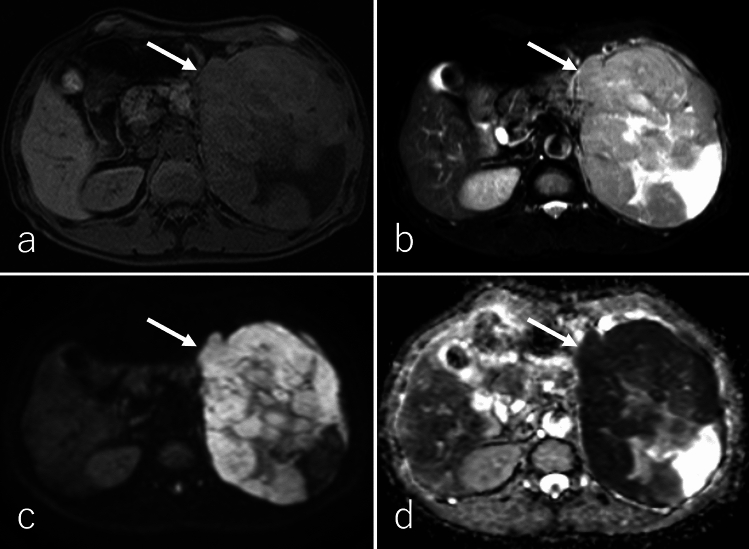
A 59-year-old male patient was diagnosed with acinar cell carcinoma (ACC). a Fat-suppressed T1-weighted imaging (FS-T1WI), b fat-suppressed T2-weighted imaging (FS-T2WI), c diffusion-weighted imaging (DWI) with *b*-value of 800 s/mm^2^, and d an apparent diffusion coefficient (ADC) map. The tumor is located in the pancreatic tail (arrow), measuring 15.0 cm in diameter, and exhibits expansile growth with well-defined margins. The tumor demonstrates heterogeneous hypointensity on FS-T1WI and heterogeneous iso- to hyperintensity on FS-T2WI and DWI. The solid region of the tumor shows lower ADC values (0.64 × 10⁻^3^ mm^2^/s), while the necrotic region exhibits higher ADC values (3.29 × 10⁻^3^ mm^2^/s) compared to the pancreatic parenchyma
